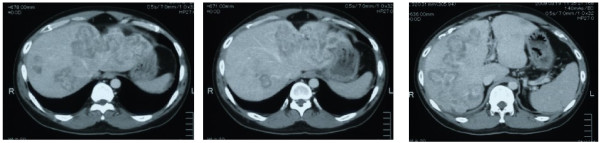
Computed tomography demonstrated expanding multiple liver metastases of the sigmoid colon cancer after FOLFIRI and FOLFOX6 therapy.
